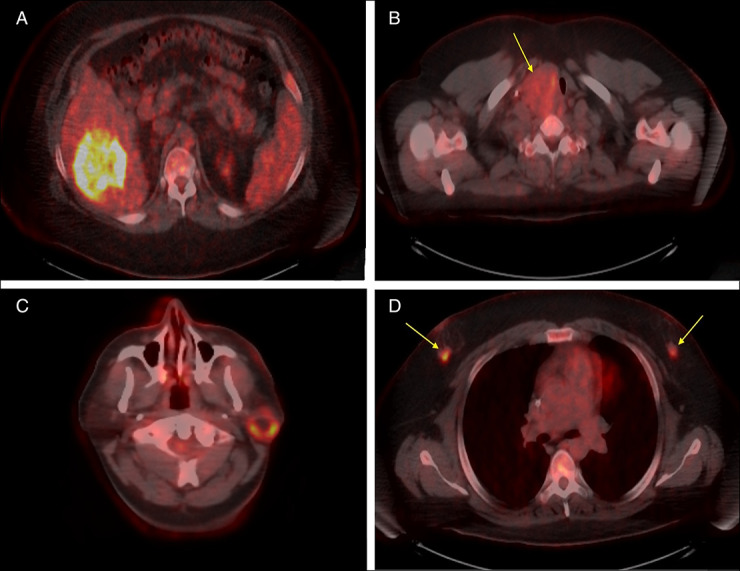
Positron emission tomography-computed tomography images of (A) solitary liver mass, (B) enlarged right thyroid gland, (C) left parotid gland, and (D) bilateral breast tissue.
